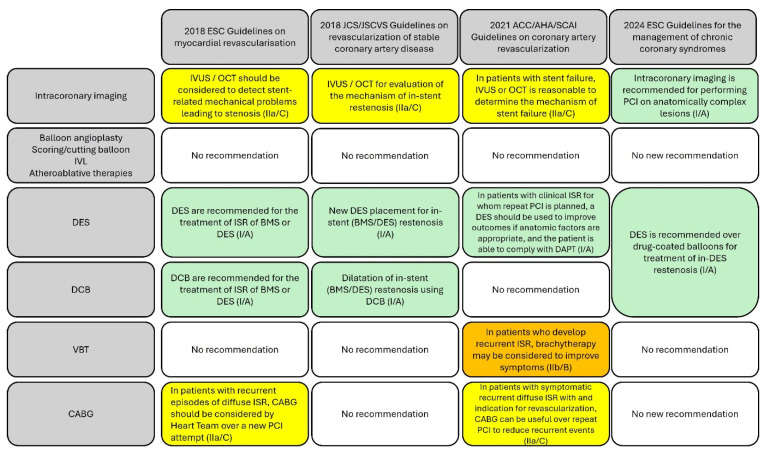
Guidelines recommendations for the management of ISR. This figure summarises the guidelines from the ESC, ACC/AHA/SCAI, and JCS/JSCVS regarding ISR management. Common key recommendations include the use of intravascular imaging, and support the use of DCB and DES. (I/A), class I recommendation and level of evidence A. Multiple class/level combinations are depicted in this figure. The respective colours represent the class recommendation; Green, class I recommendation; Yellow, class IIa recommendation; Orange, class IIb recommendation; ACC, American College of Cardiology; AHA, American Heart Association; BMS, bare-metal stent; CABG, coronary artery bypass graft; DCB, drug-coated balloons; DES, drug-eluting stent; ISR, in-stent restenosis; IVL, intravascular lithotripsy; IVUS, intravascular ultrasound; JCS, Japan Circulation Society; JSCVS, Japanese Society for Cardiovascular Surgery, OCT, optical coherence tomography; PCI, percutaneous coronary intervention; SCAI, Society for Cardiovascular Angiography and Interventions; VBT, vascular brachytherapy.
